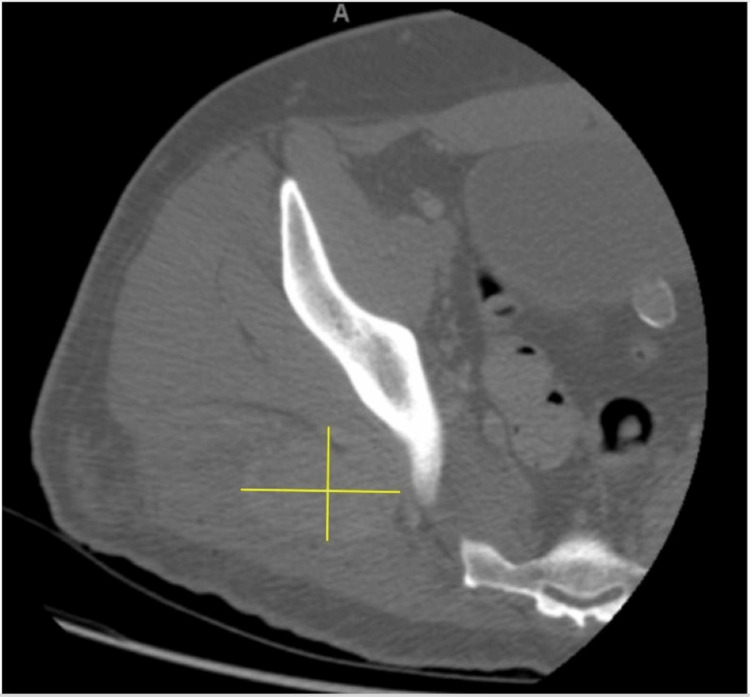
CT right lower extremity with contrast CT right lower extremity with contrast showing a heterogeneously enhancing soft tissue mass centered in the right gluteus maximus muscle measuring 6.3 x 4.0 cm (marked).
